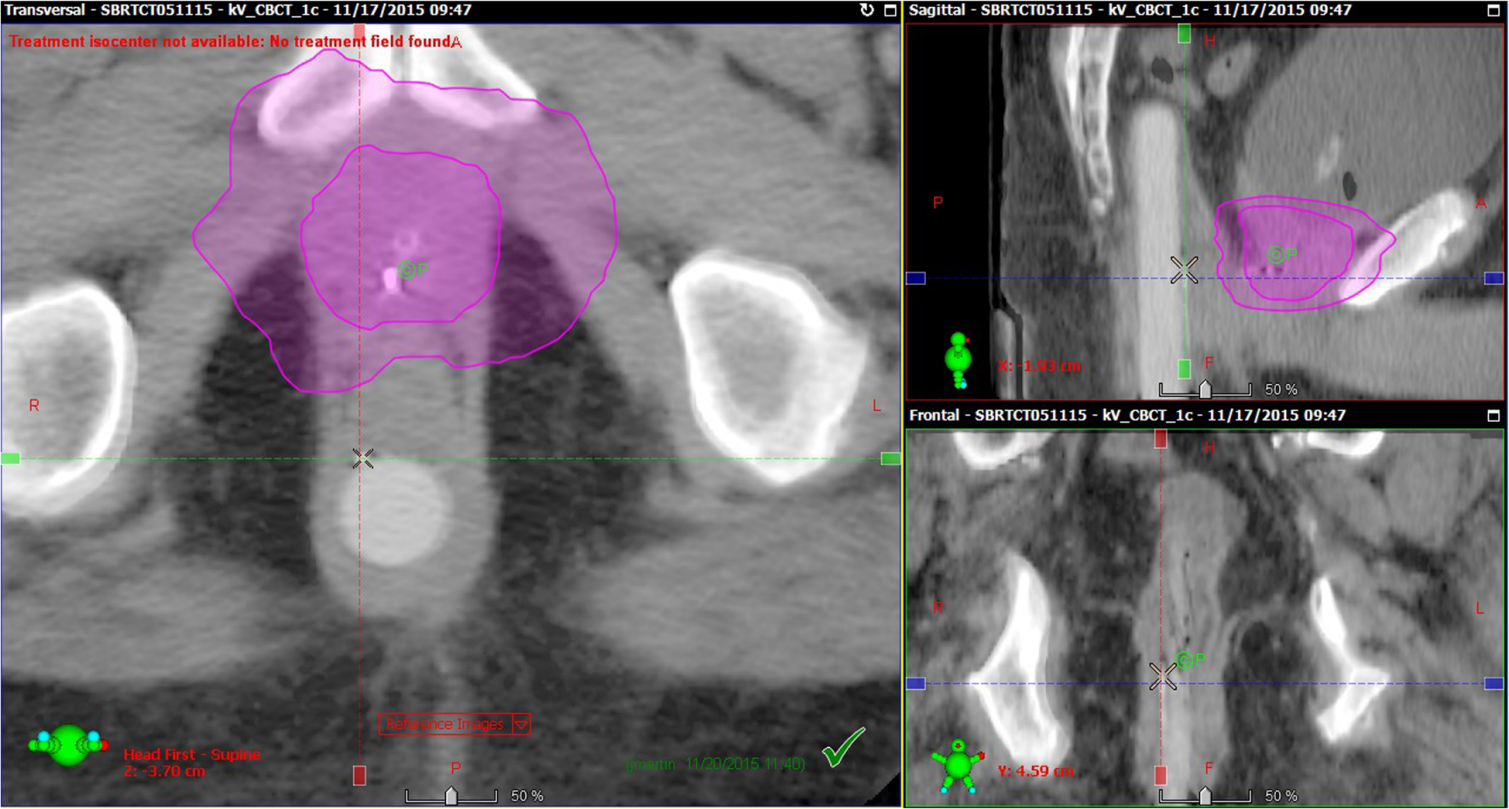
Marker at MO*Skin* location on CBCT scan taken following a patient treatment. The CTV and 50% isodose regions appear in *purple*For patients treated using a 6 MV or 10 MV beam with a flattening filter, a verification plan was created in the Eclipse treatment planning system with the treatment split into sub-arcs, each of 5.2°. The dose file for each sub-arc and the plan file were then exported from the system. For 10 MV flattening filter free beams, the version of the planning system available was unable to automatically split patient plans into sub-arcs. Five partial treatment plans were created for each of these patients, evenly splitting each arc by control points to obtain five cumulative dose points for each treatment. Following export of all dose and plan files, in-house software was used to extract the dose and gantry angle for each arc at the location of the MO*Skin* verification points. The position of the gantry with time was determined using spline interpolation from the gantry angles recorded in the header of the EPID images acquired at 15.26 Hz during treatment.Planned dose at the verification point and MO*Skin* measured dose were then plotted as a function of time over the whole course of each treatment fraction. The root mean square error (RMSE) was found for each treatment fraction to compare the MO*Skin* measured dose with the planning system dose across the whole treatment time. The RMSE value was normalised to the final planning system dose for each fraction to enable comparison between different patient plans.
